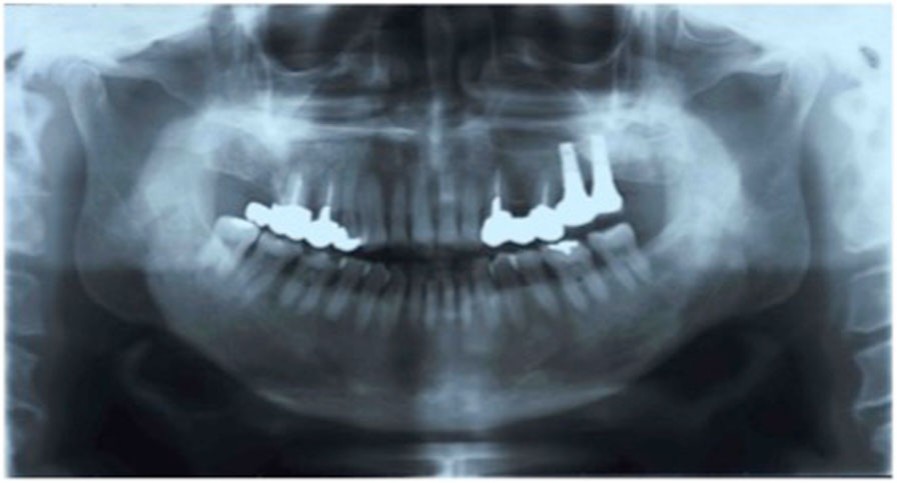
Nine-year follow-up radiograph of the implants
